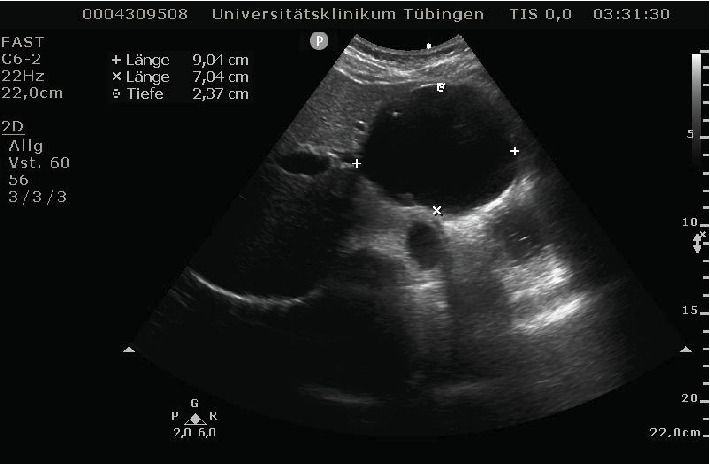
Sonography of the abdomen showing a cystic lesion in the liver (dimensions: 9 × 7 cm).
